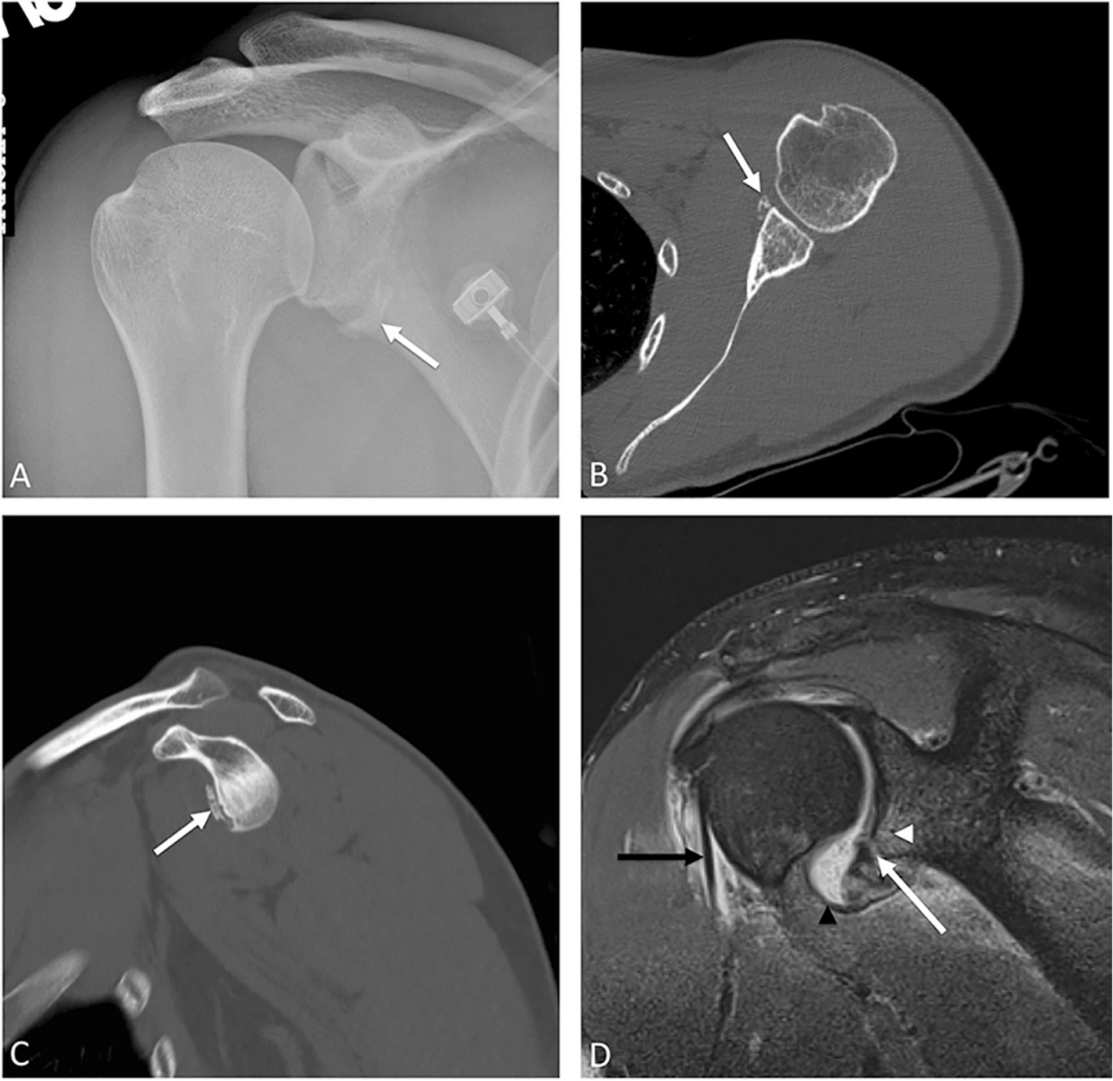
Bony Bankart on radiograph (a), CT (b, c), and MRI (d) in several patients. a Crescentic bone fragment at the inferomedial aspect of the glenohumeral (GH) joint space (*white arrow*) in this young man who was post anterior dislocation and reduction. This is a medially displaced bony Bankart fracture. Axial (b) and sagittal CT (c) with typical appearance of anterior glenoid fracture (*white arrows*). d Oblique coronal proton density fat-saturated MR shows inferior glenoid marrow edema (*arrowhead*) and bony Bankart fracture (*arrow*). Joint effusion is present, with distention of the inferior GH capsule (*black arrowhead*). Long head biceps tendon is surrounded by fluid (*black arrow*), which tracks in the biceps sheath—and extension of the articular space
